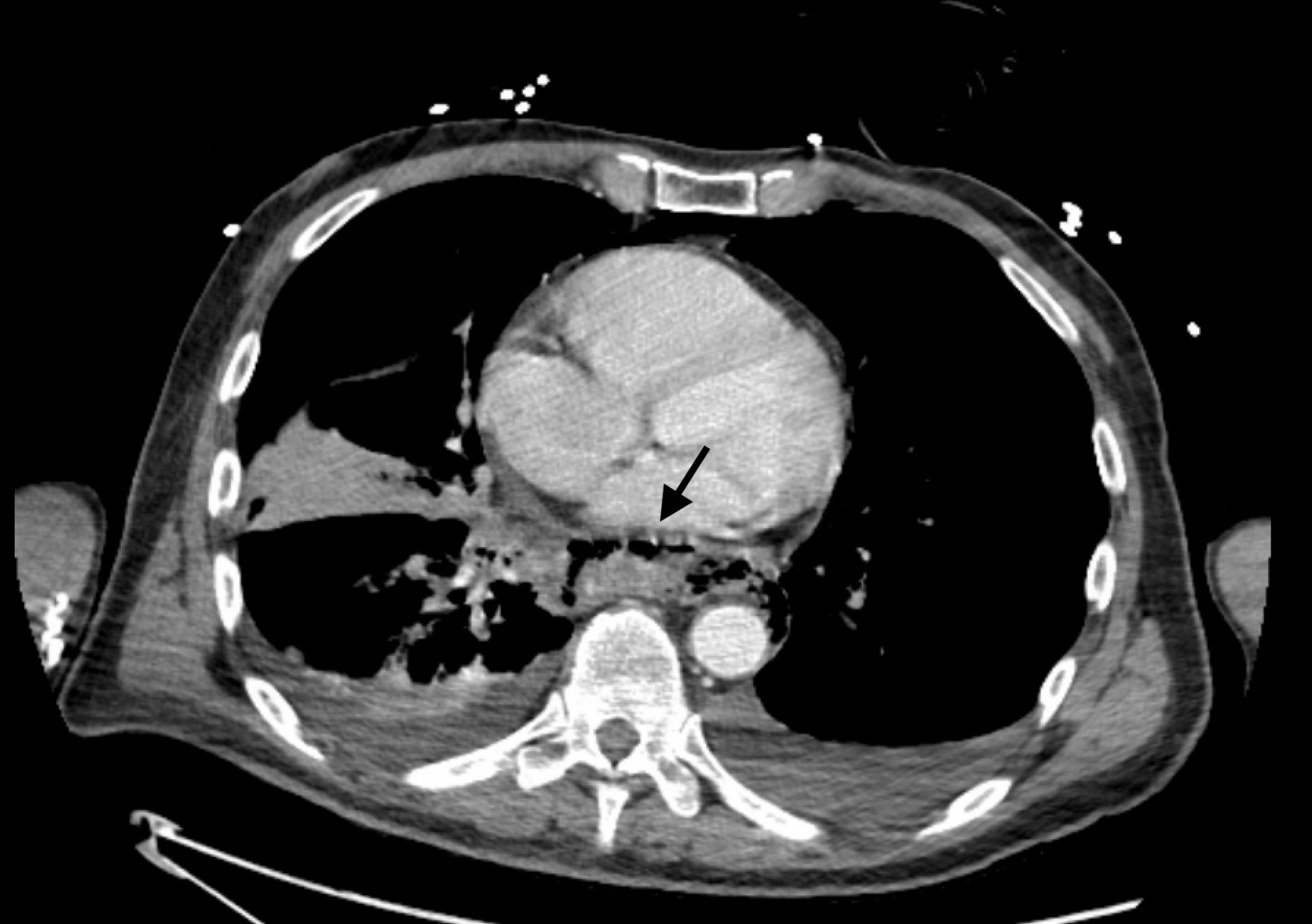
Axial CT of the chest with intravenous contrast demonstrated pneumomediastinum with extraluminal air tracking along the distal esophagus and mediastinal fat stranding, consistent with esophageal perforation. A gas-containing collection was seen adjacent to the esophagus, suggestive of a walled-off leak or developing abscess.
